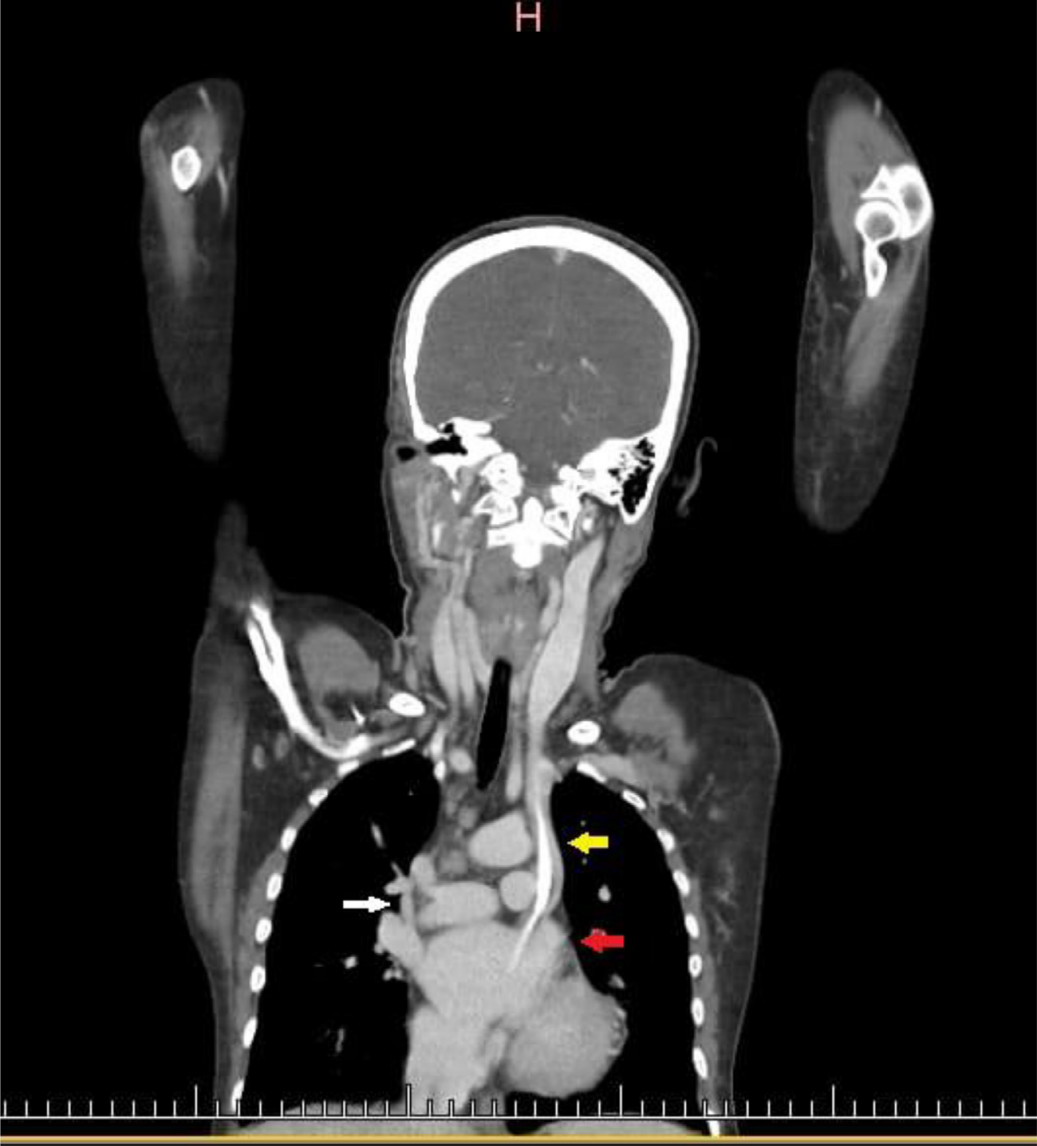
Coronal view of CT scan (White arrow pointing at right SVC, Yellow arrow pointing at PLSVC, Red arrow pointing at left atrium).
